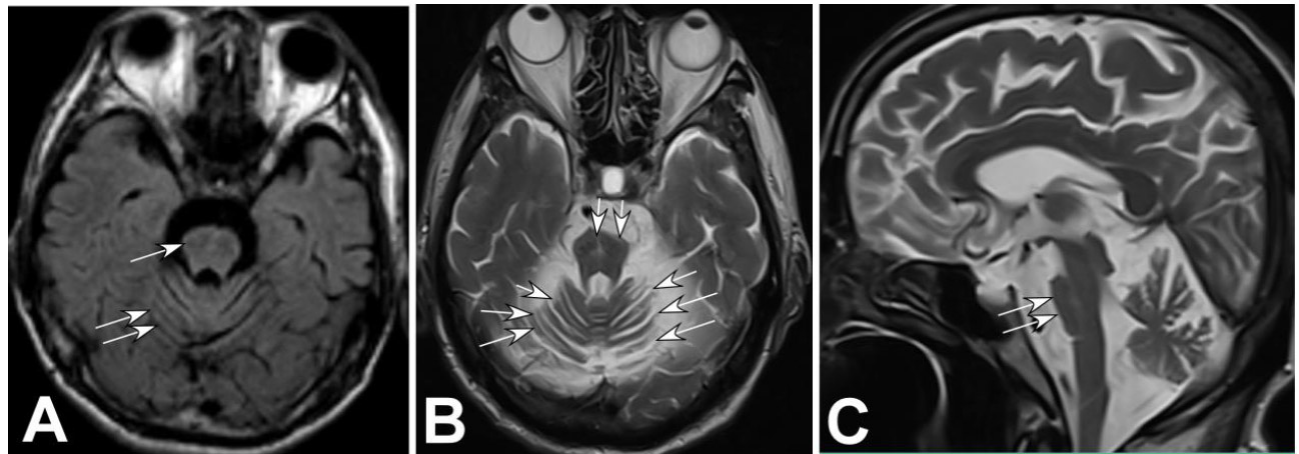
Magnetic resonance imaging (MRI) of the brain in case 1. (A). FLAIR sequence (A) showed cerebellar atrophy (double arrows) and thinning of the brainstem (arrow). (B). The top view demonstrates cerebellar atrophy (arrows) and thinning of the brainstem (double arrows). (C). Lateral view demonstrated decreased signal at the anterior edge (double arrows) of the pons.
